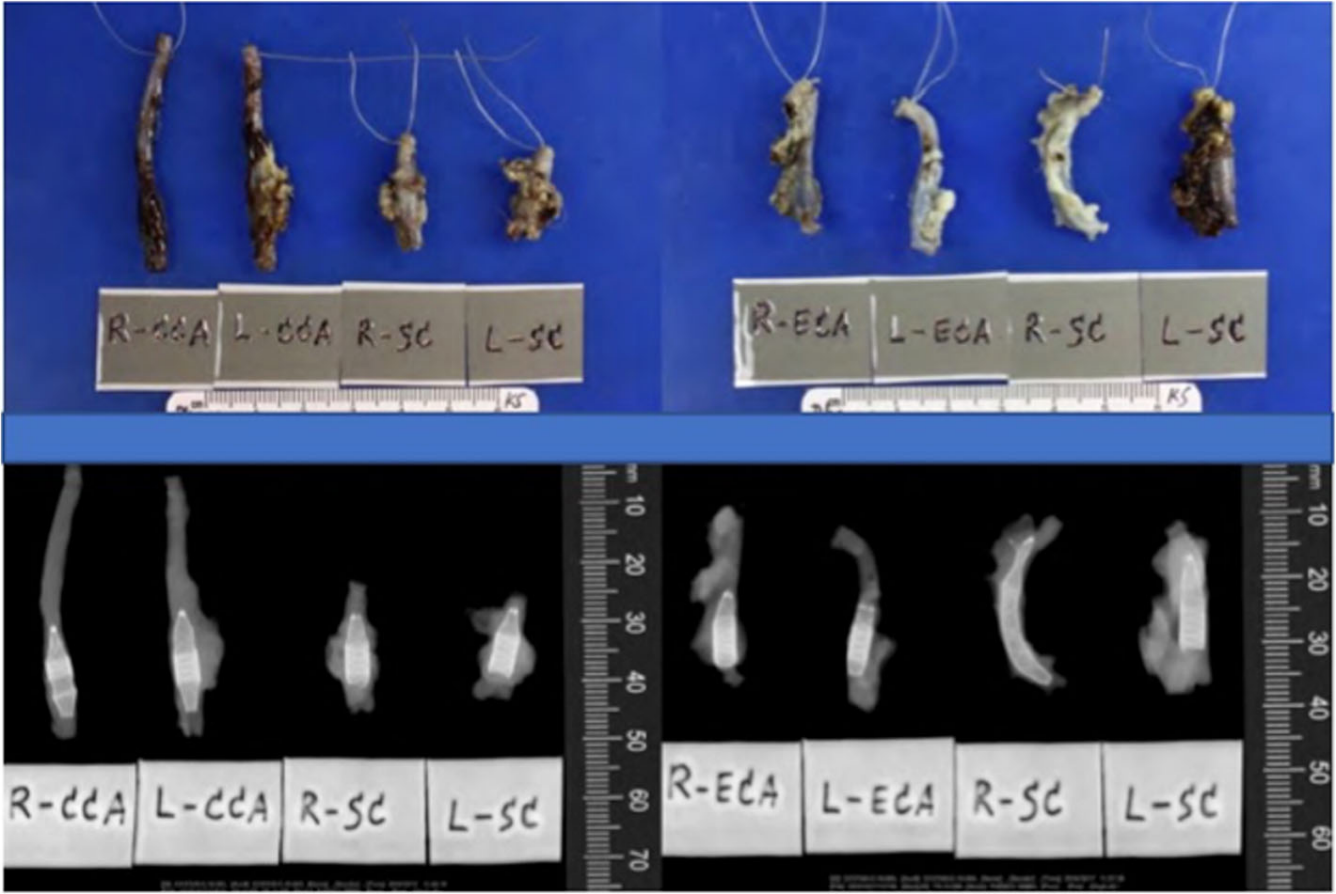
Macroscopic and radiographic analysis of the explanted vessel segments of subjects number 1 and 2
